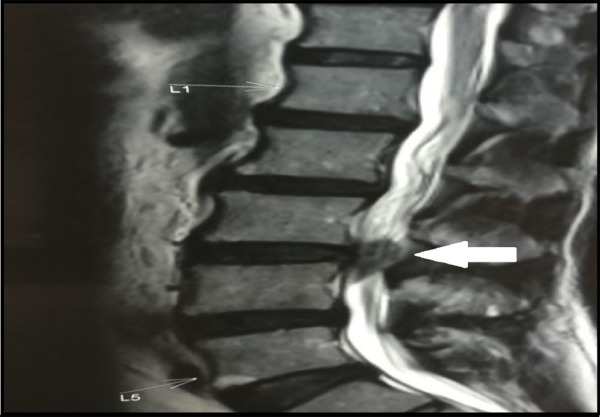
Preoperative 3 Tesla MRI lumbar spine T2 weighted sagittal section.
